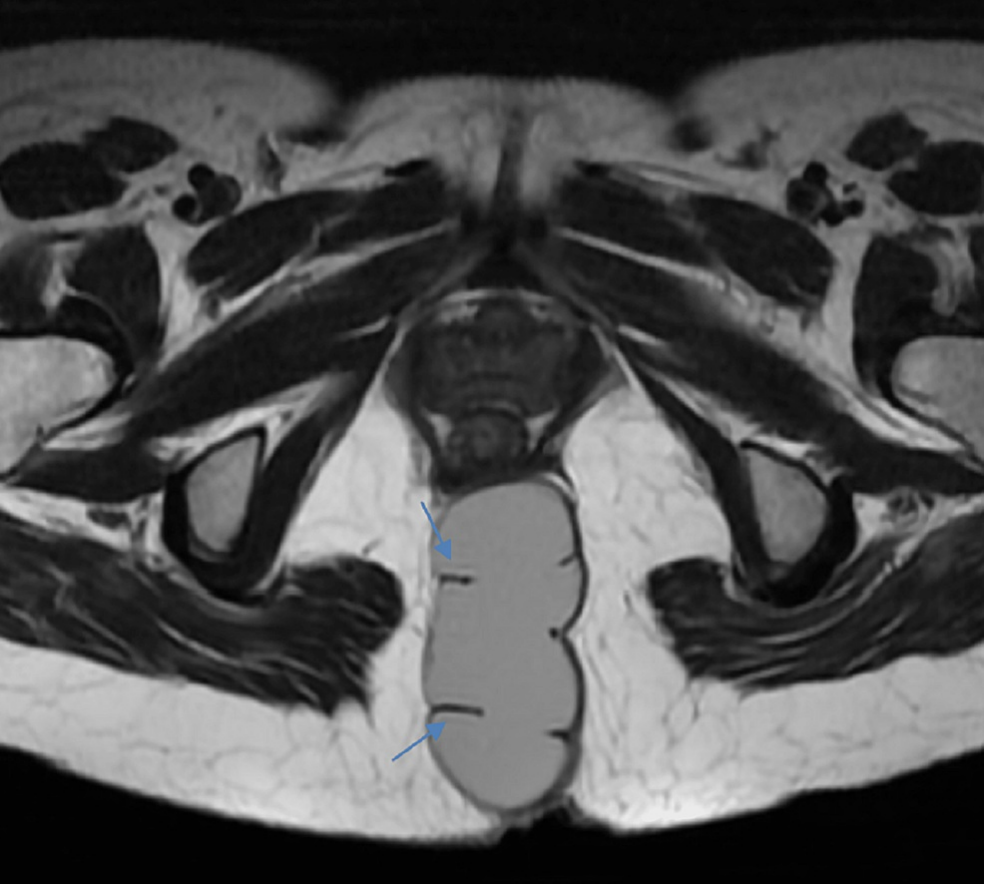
Axial MRI T2WI images showing well-defined hyperintense lesions showing incomplete septations within (blue arrow). T2WI: T2 weighted image https://assets.cureus.com/uploads/figure/file/450603/lightbox_010afed0313c11ed8339570870798b63-E4.png
